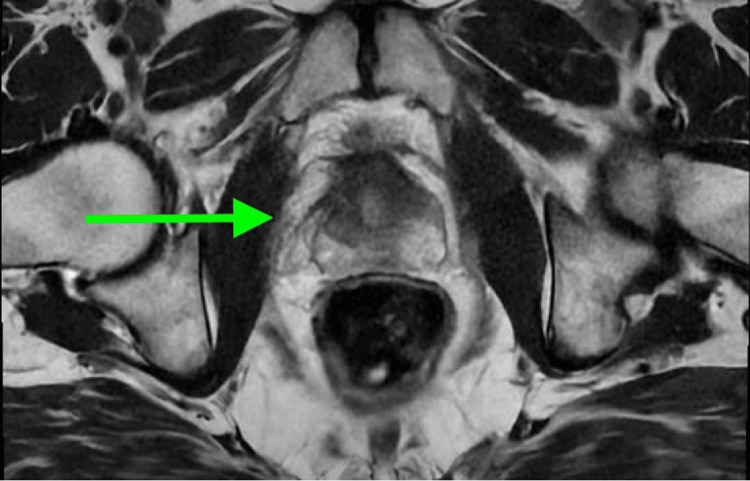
Multiparametric Prostate Magnetic Resonance Imaging (MRI), Axial T2-Weighted Image, Demonstrating No Prostate Imaging Reporting and Data System (PI-RADS) Score ≥3 Lesion No focal lesion meeting PI-RADS ≥3 criteria, suggesting no imaging evidence of clinically significant prostate cancer. The arrow points to the prostate gland.
